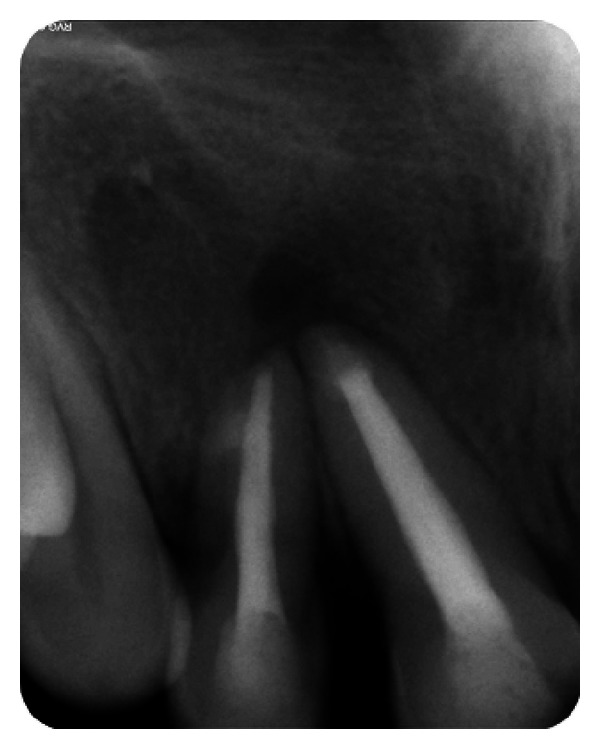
6-month followup IOPA radiograph.
